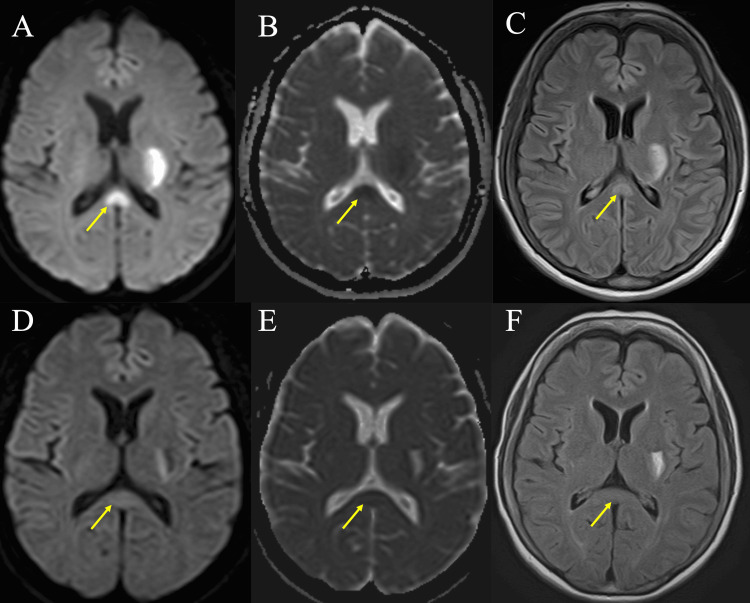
Case 6: MRI findings of CLOCCs (A) DWI on day 7 shows a high-signal-intensity lesion in the corpus callosum (yellow arrow). The initial MRI showed left putamen and corona radiata infarction, which is not shown. (B) The ADC map shows corresponding low signal intensity (yellow arrow). (C) FLAIR imaging shows high signal intensity in the corpus callosum (yellow arrow). (D-F) Follow-up MRI shows complete resolution of the corpus callosum lesion on all sequences. ADC, apparent diffusion coefficient; CLOCCs, cytotoxic lesions of the corpus callosum; DWI, diffusion-weighted imaging; FLAIR, fluid-attenuated inversion recovery
